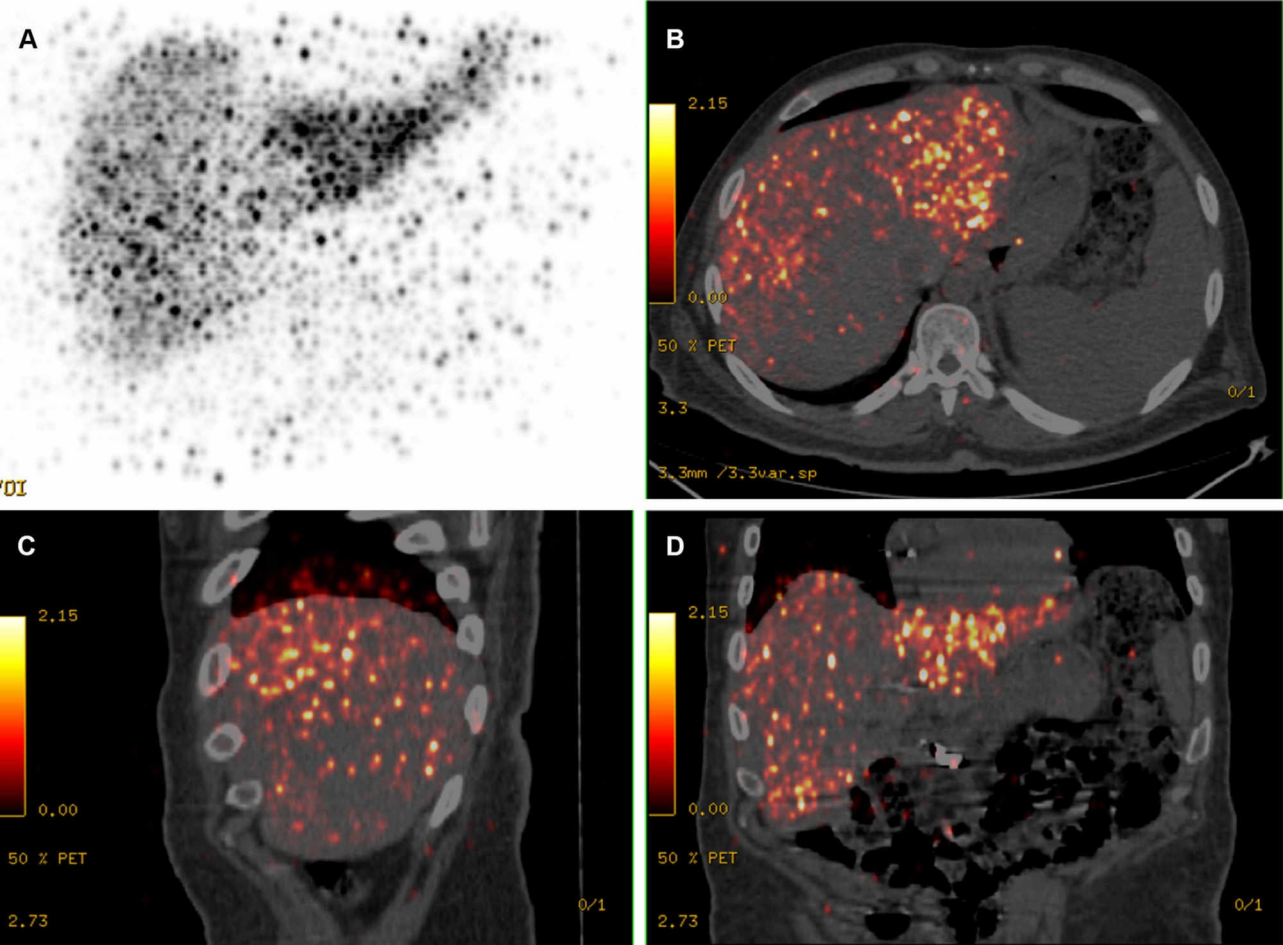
Y-90 positron emission tomography–computed tomography (PET/CT) imaging Following radioembolization, Y-90 positron emission tomography–computed tomography (PET/CT) imaging shows non-homogeneous distribution of Y-90 microspheres inside the hepatic parenchyma, as revealed on the 3D PET (A) image and the PET/CT fusion transverse (B), sagittal (C), and coronal (D) views.
